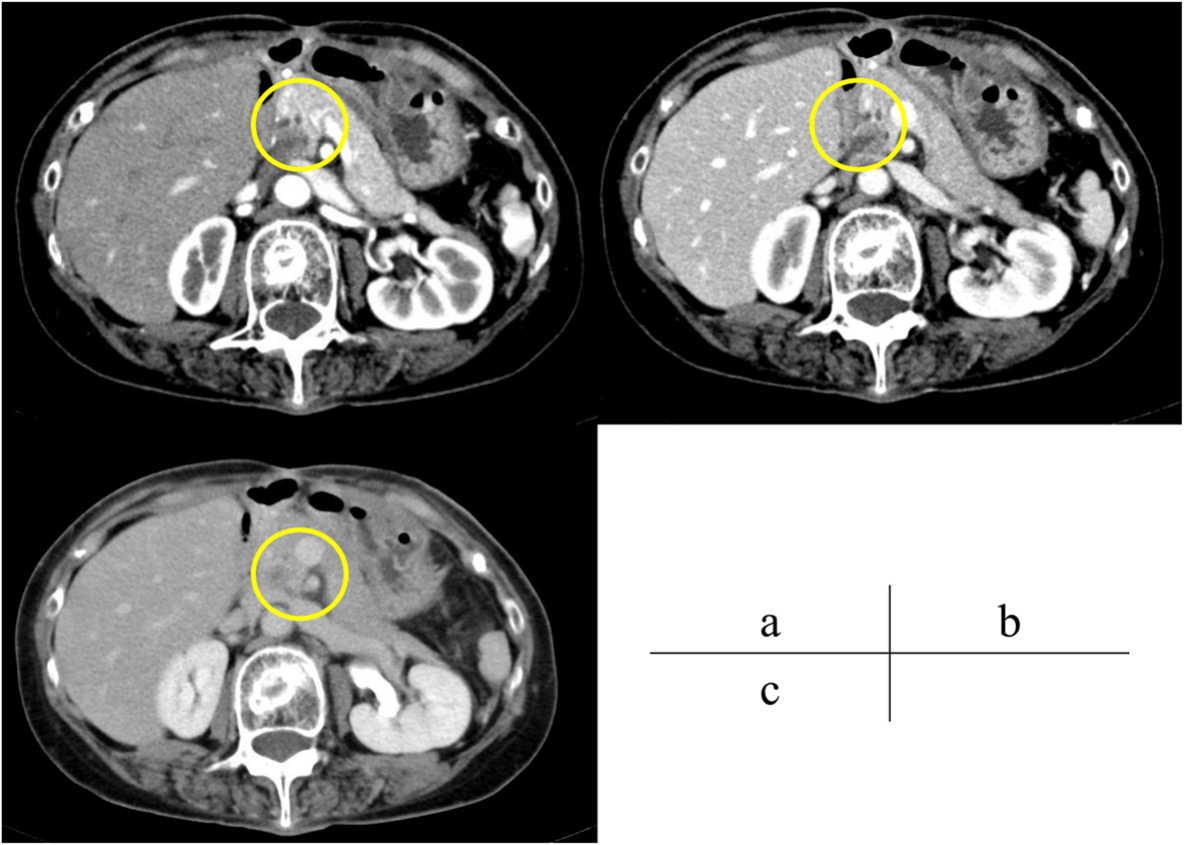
Dynamic abdominal computed tomography (CT) demonstrating a slightly enhanced round tumor at the pancreatic head (a, yellow circle). Tumor enhancement was evident from the portal phase to the equal phase (b, c)
